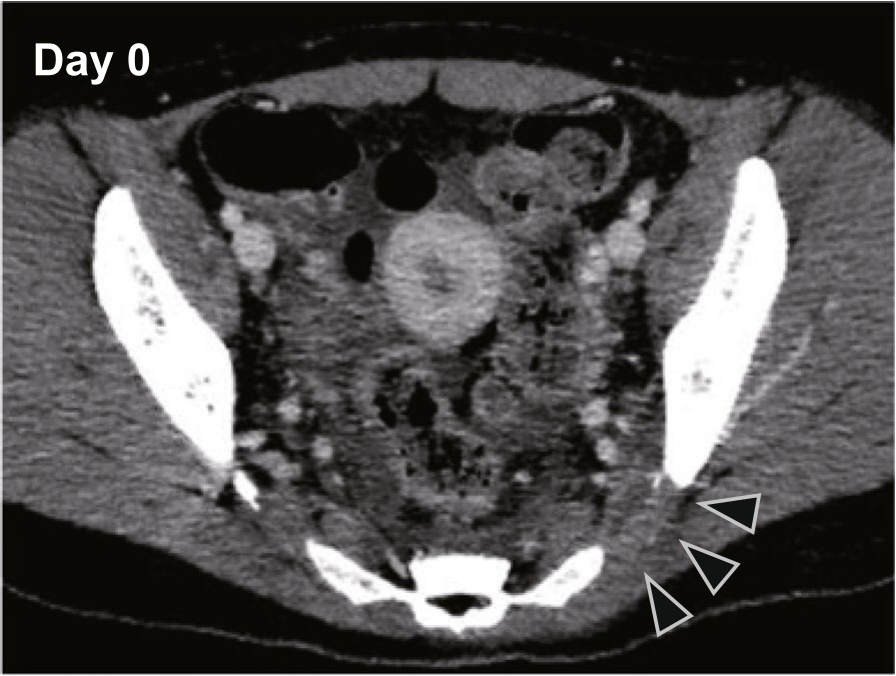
Pelvic computed tomography image upon admission. The arrowhead indicates fluid collection at the left sacroiliac joint
